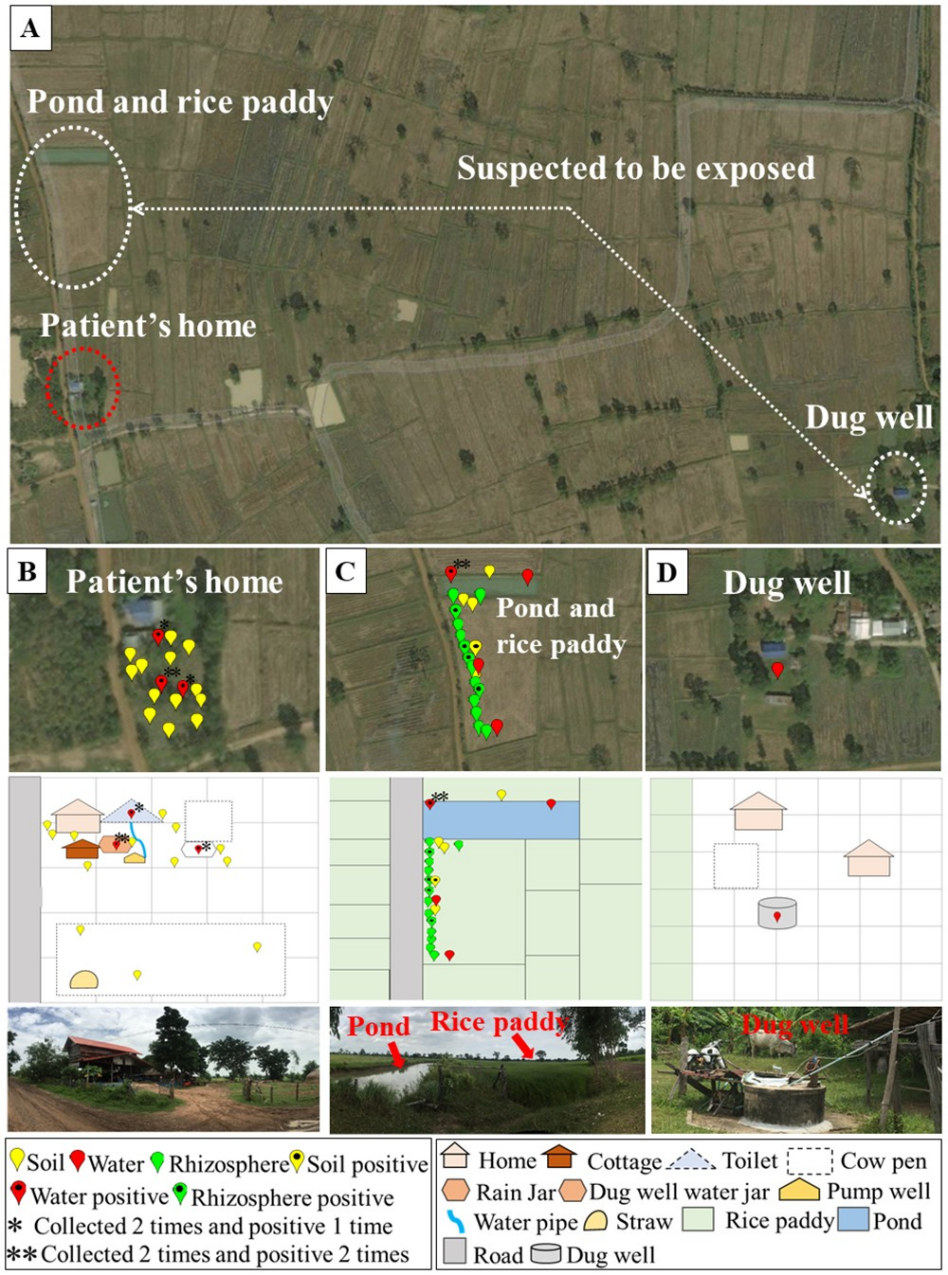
Geographic location of the sampling sites and points. (A) The locations at which the patient was suspected to be exposed (suspected to be exposed), the patient’s house (patient’s house) and the community-dug well used by the patient and community for drinking and cooking (dug well). (B) The pins denote the sampling points for the locations near the patient’s house. (C) The pins denote the sampling points around the pond and the rice paddy field to which the patient was suspected to be exposed. (D) The pins denote the water samples and the enclosed area surrounding the dug well. The sampling points were tracked using GPSMAP 60CSx, and the map was created using LandsatLook Viewer (http://landsatlook.usgs.gov/). Pins with black spots represent *B*. *pseudomallei* culture-positive samples.
